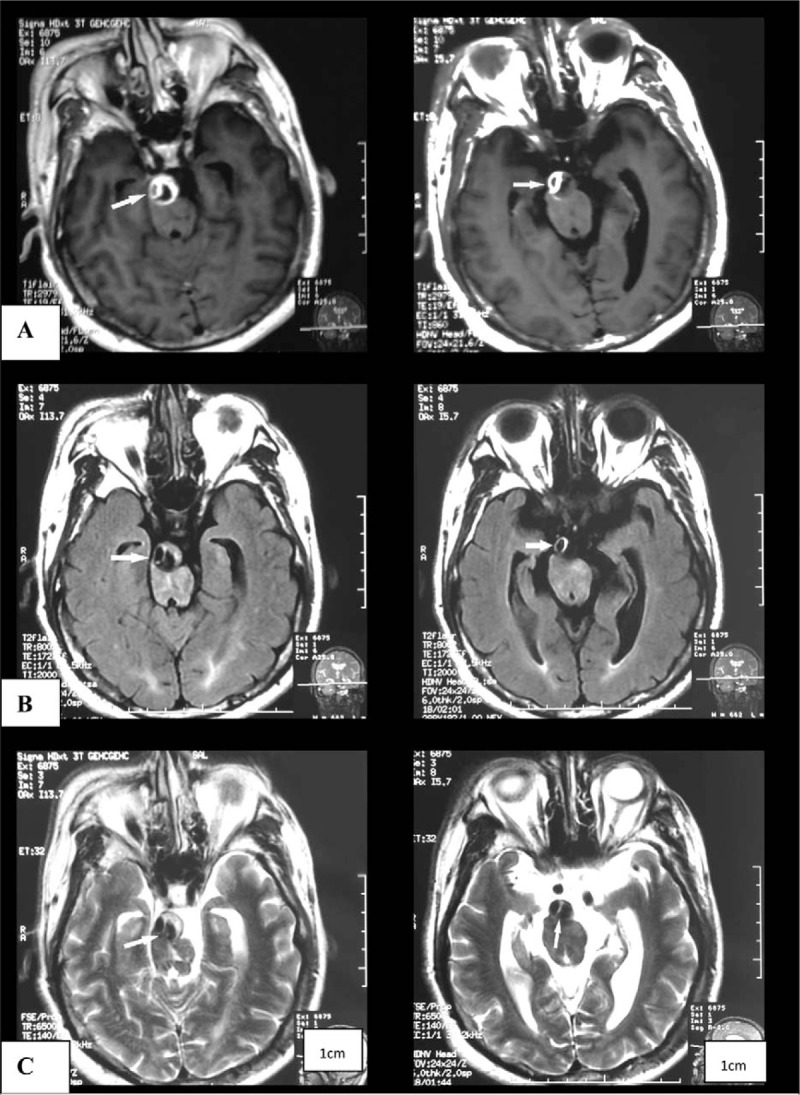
“Two lumen” sign on brain MRI, 1 is in crescent shape: (A) T1 flair; (B) T2 flair; and (C) weighted imaging. MRI = magnetic resonance imaging.
